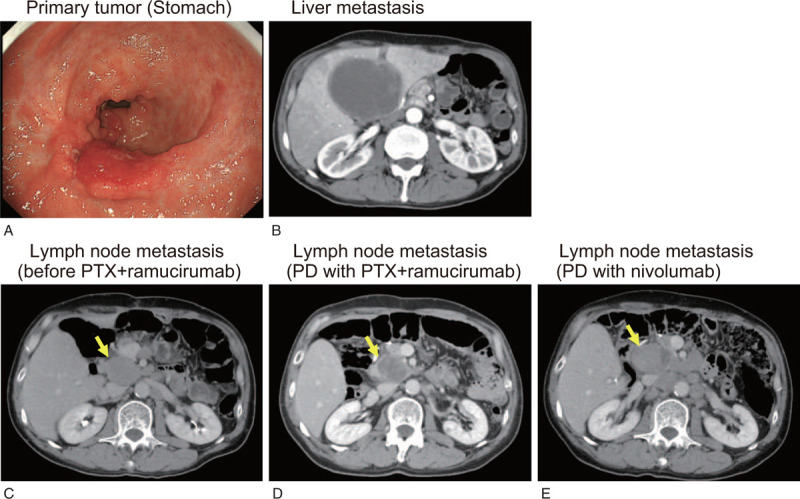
Clinical images during course of treatment. (A) Upper gastrointestinal endoscopy image at diagnosis. A Borrmann type 3 tumor that protruded was located at the pyloric antrum. (B) A computed tomography (CT) image of liver metastasis. The tumor located at the liver S4 lesion increased in size, and its internal area with poor contrast was enlarged. This lesion was suspected to be complicated by an abscess. (C-E) CT images of lymph node metastasis at the time before paclitaxel (PTX) plus ramucirumab therapy (C), disease progression after PTX plus ramucirumab therapy (D), and disease progression after nivolumab therapy (E). Metastatic lymph nodes (yellow arrows) remarkably increased in size after nivolumab therapy (c-e).
